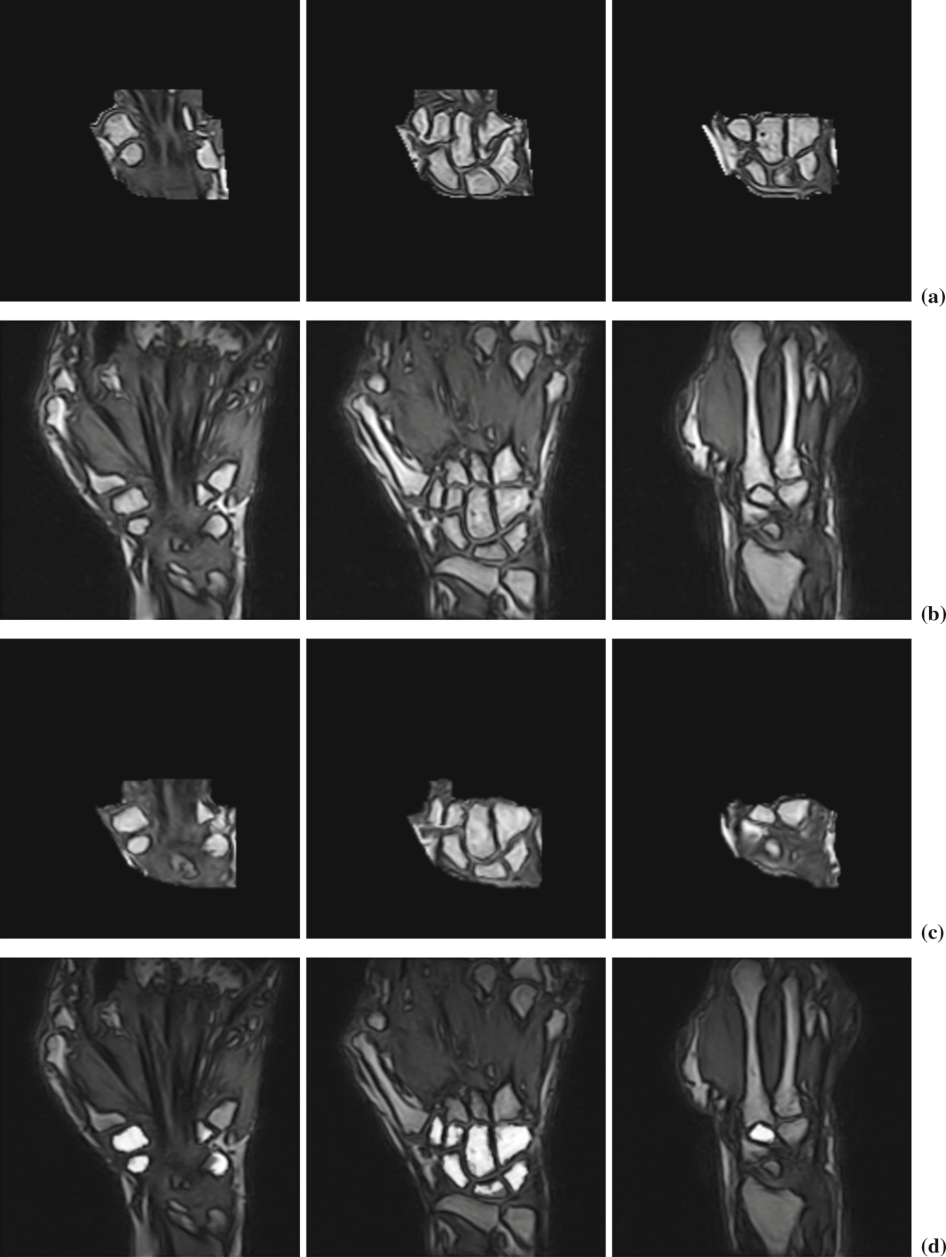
Coronal slices from MR images of wrist: a an atlas image, b a sample image, c and atlas image registered atlas to a sample image, d atlas markers transformed to the space of the sample image
